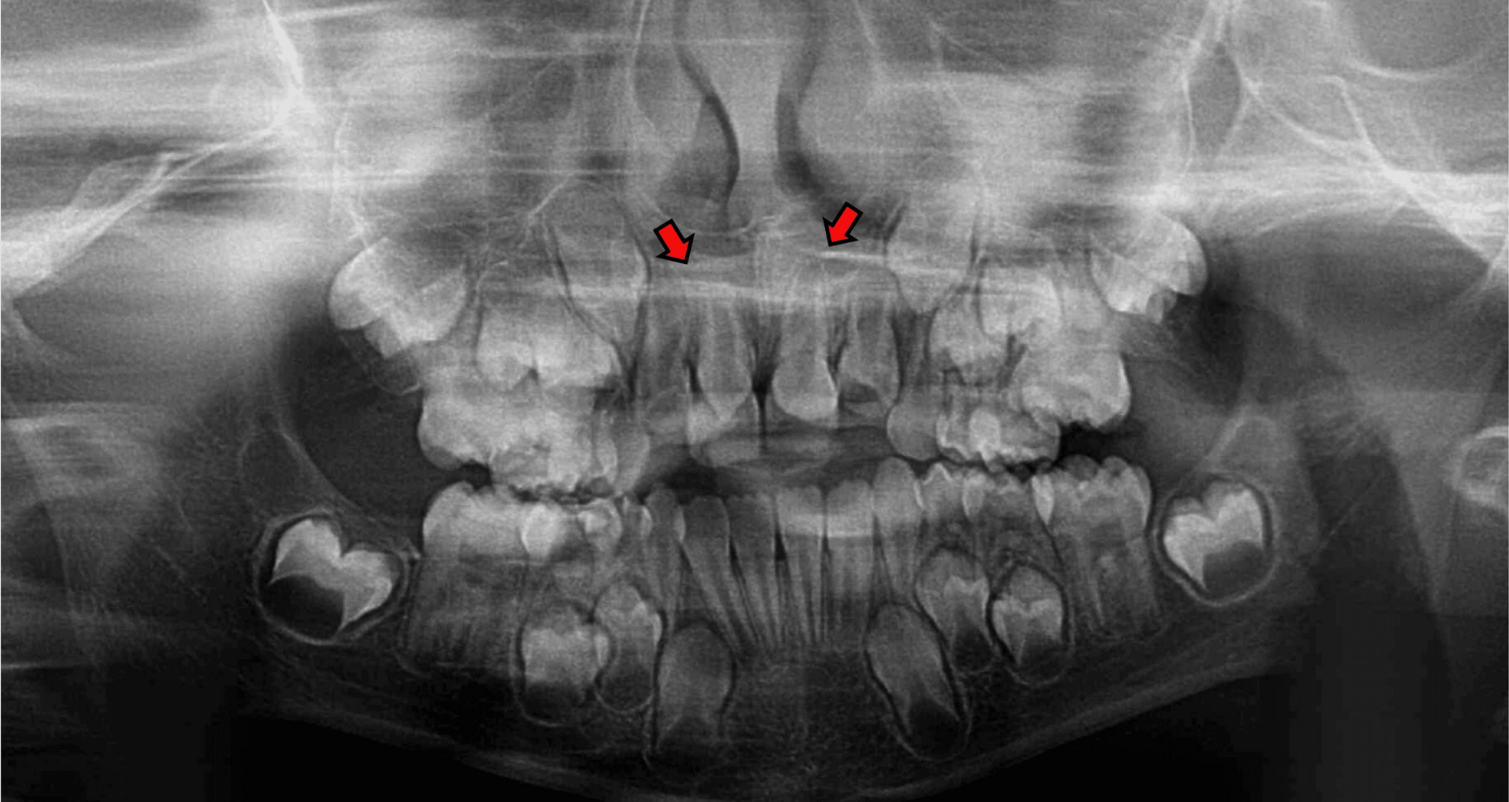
Panoramic radiograph The red arrows highlight the presence of two impacted supernumerary teeth located in the anterior maxillary region, positioned palatally to the permanent central incisors.
